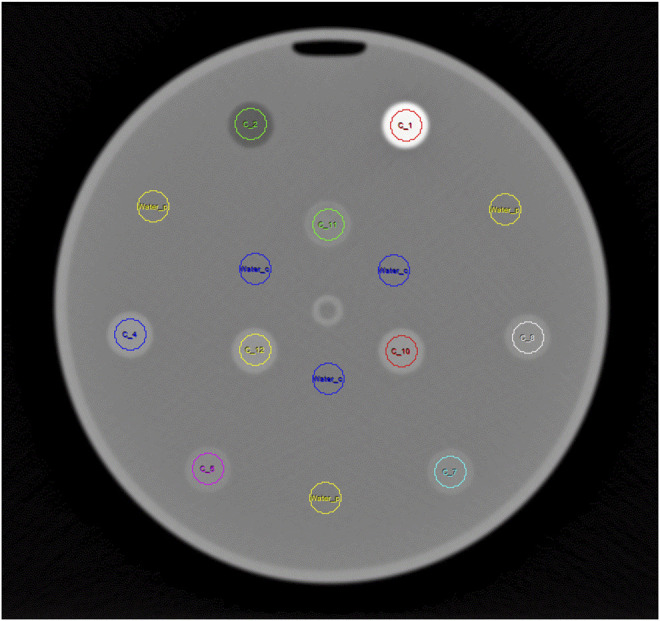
Sample CT slice showing ROIs used for HU measurement in the printed cylinders arranged in two circles. Additional ROIs used to measure HU in the water are shown in blue (inner circle) and yellow (outer circle).
